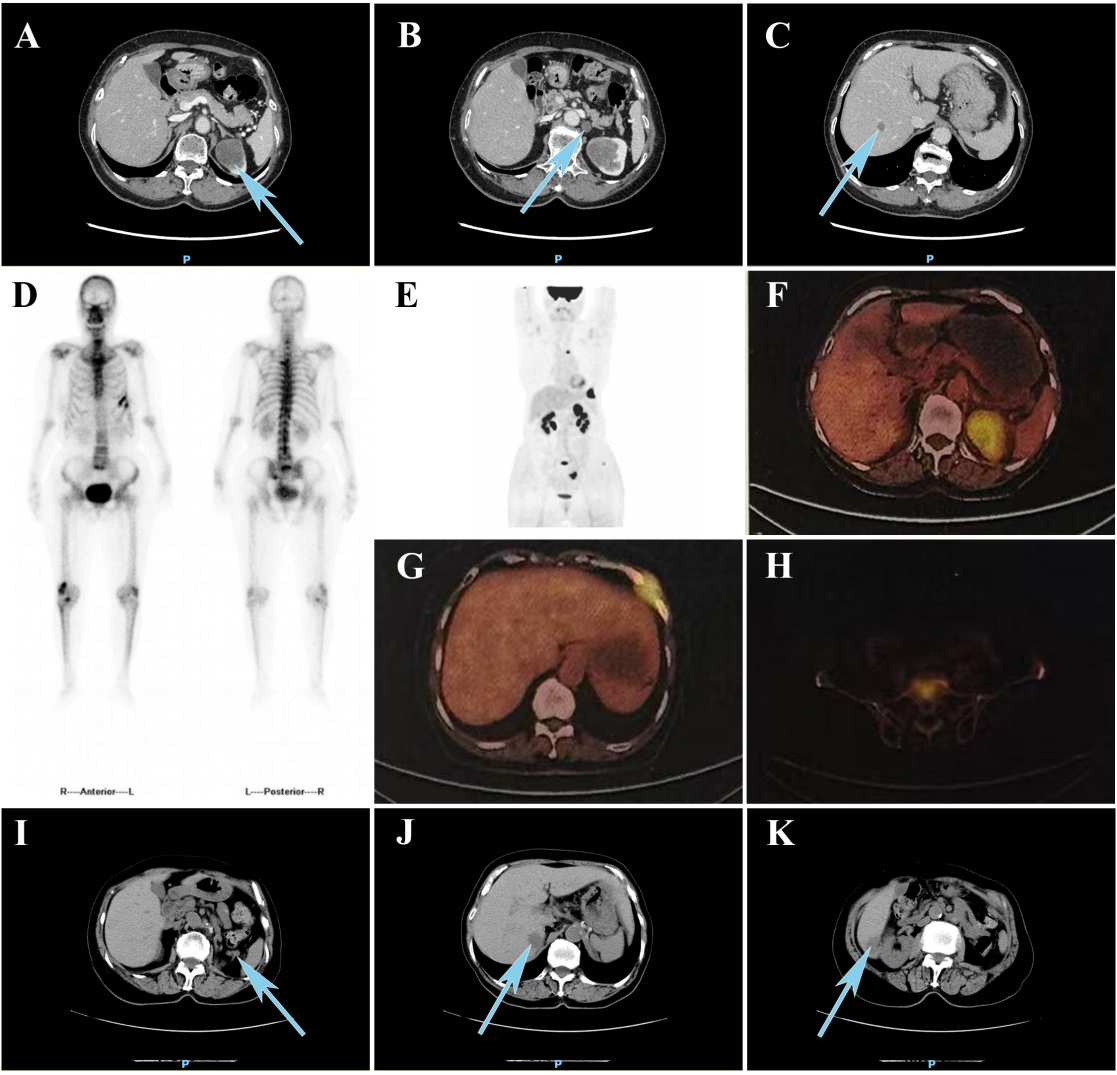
(A) Contrast-enhanced CT reveals (A) a heterogeneously enhancing mass at the left renal upper pole, (B) progressive retroperitoneal lymphadenopathy, and (C) hypodense lesions in the liver. (D)^99m^Tc-MDP bone scintigraphy demonstrated increased tracer uptake in the left sixth and seventh anterior ribs, sacrum, and left sacroiliac joint. (E) MIP reconstruction of ^18^F-FDG PET/CT. ^18^F-FDG PET/CT demonstrated increased fluorodeoxyglucose uptake in (F) the upper pole of the left renal upper pole, (G) left sixth and seventh ribs, and (H) L3 vertebral body. Follow-up CT at 3 months demonstrated newly (I) enlarged retroperitoneal lymph nodes and (J, K) two hypoattenuating hepatic lesions. CT, computed tomography; 99mTc-MDP, technetium-99m methylene diphosphonate; MIP, maximum intensity projection; ^18^F-FDG, fluorine-18 fluorodeoxyglucose; PET, positron emission tomography.
